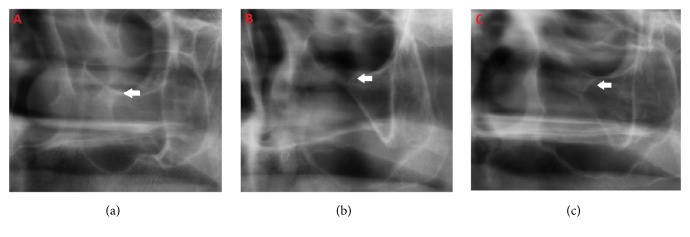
The cropped panoramic radiographic images show the types of infraorbital canals: (a)* Type I*, corticated both orbit and antrum parts corticated, (b)* Type II*, both orbit and antrum parts without cortication, and (c)* Type III*, orbital part without cortication, antrum part corticated.
